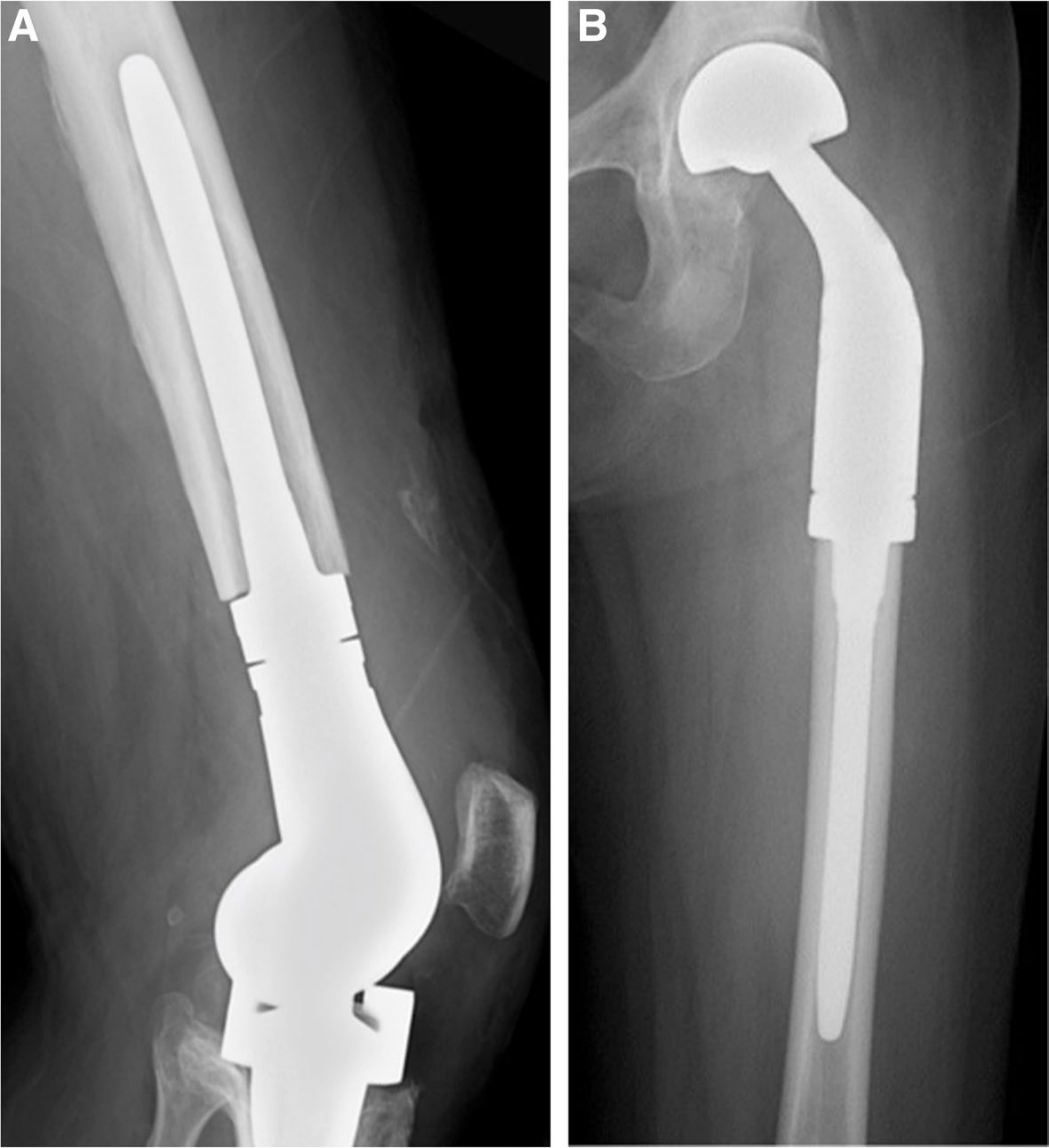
A 3-year postoperative radiograph showing the lateral view of the KMLS newly designed cementless stem in a patient with A. giant bone cell tumor and B. bone metastases from breast cancer. KMLS, Kyocera Modular Limb Salvage System
